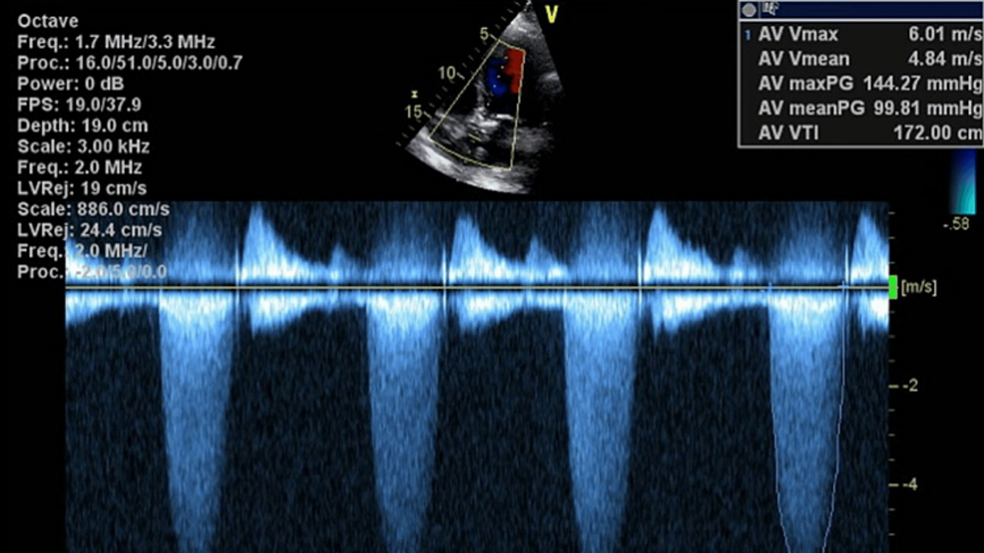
Transthoracic echocardiogram showing the aortic peak velocity of 6.1 m/s, the highest AV mean gradient of 99.8 mmHg, and the AV area calculated at 0.37 cm². The AV velocity ratio/Dimensionless Index is 0.14. There is severe prosthetic aortic stenosis. AV: Aortic valve.
